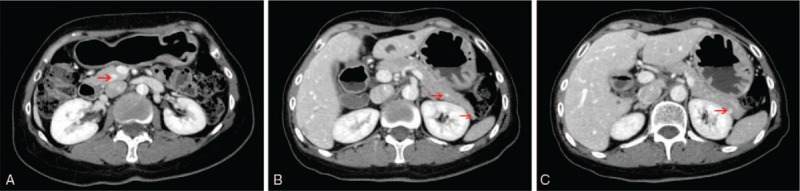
Preoperative abdominal computed tomographic (CT) scan. A: One local thickening in the descending duodenum wall (red arrows); B: Several lesions located in the head, body and tail of pancreas (red arrows).
